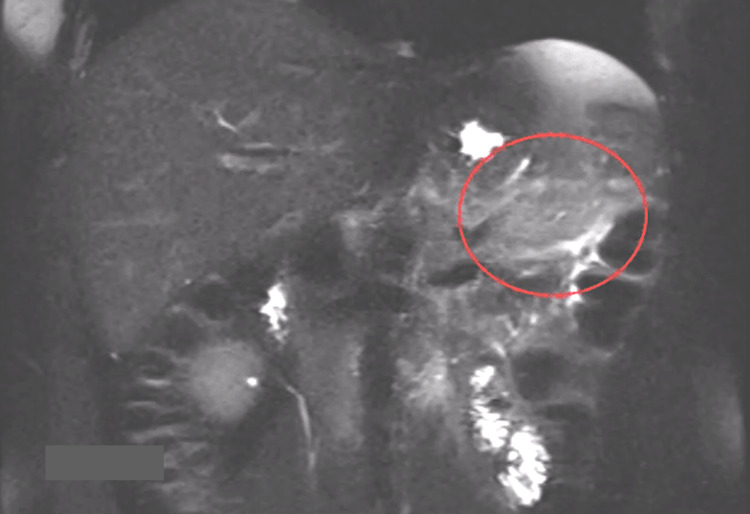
Coronal T2-weighted with fat-sat showing peripancreatic edema (red circle) Fat-sat: fat-saturation.
